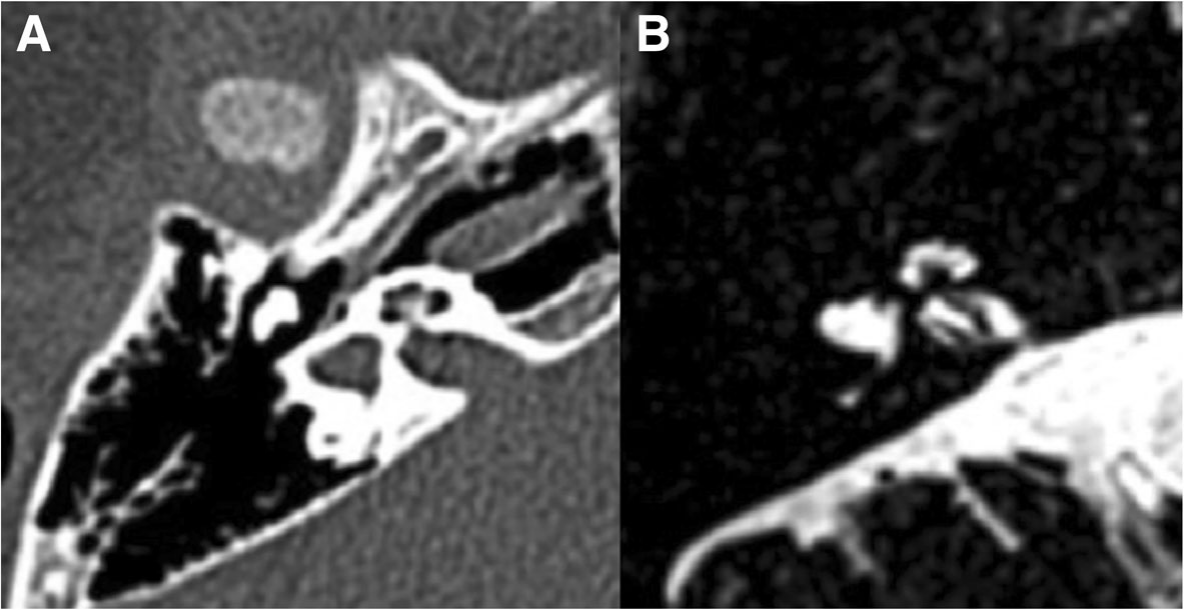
Temporal bone CT (a) and MRI (axial T2/3D) (b) from a 2-year-old patient with Down syndrome at the level of the internal auditory canal, showing complete absence of the right bone island and consequent aplasia of the lateral semi-circular canal. Note also the dysplastic vestibule as a result of the absence of the lateral semi-circular canal
